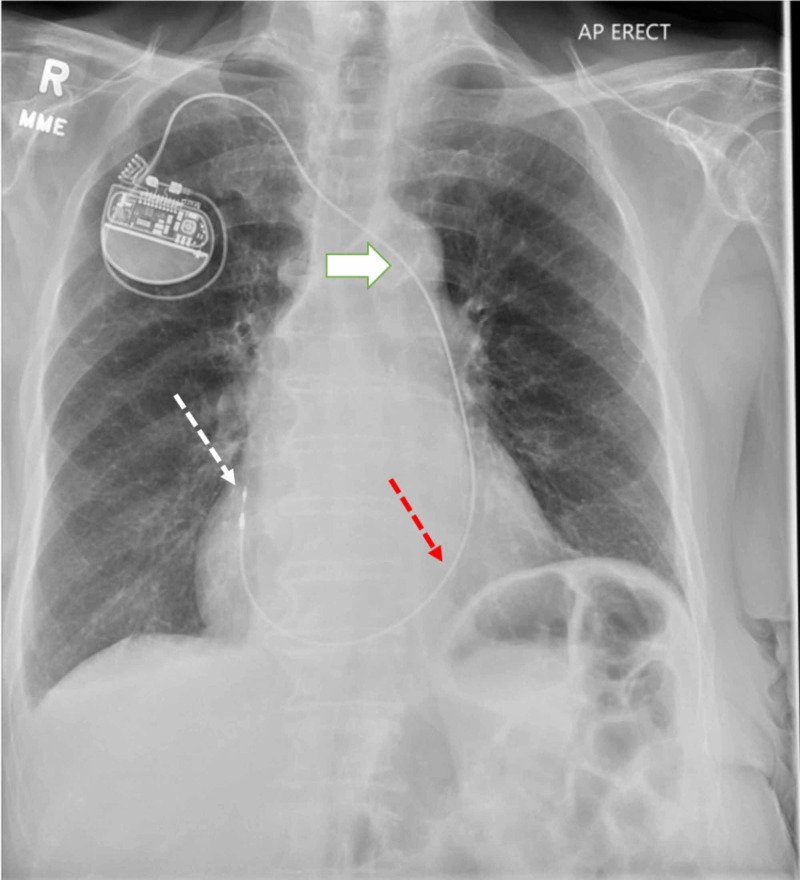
Chest radiograph with a pacer lead coursing through LSVC (white solid arrow), CS (red dotted arrow), and terminating in RA (white dotted arrow) CS: coronary sinus, LSVC: left superior vena cava, RA: right atrium
